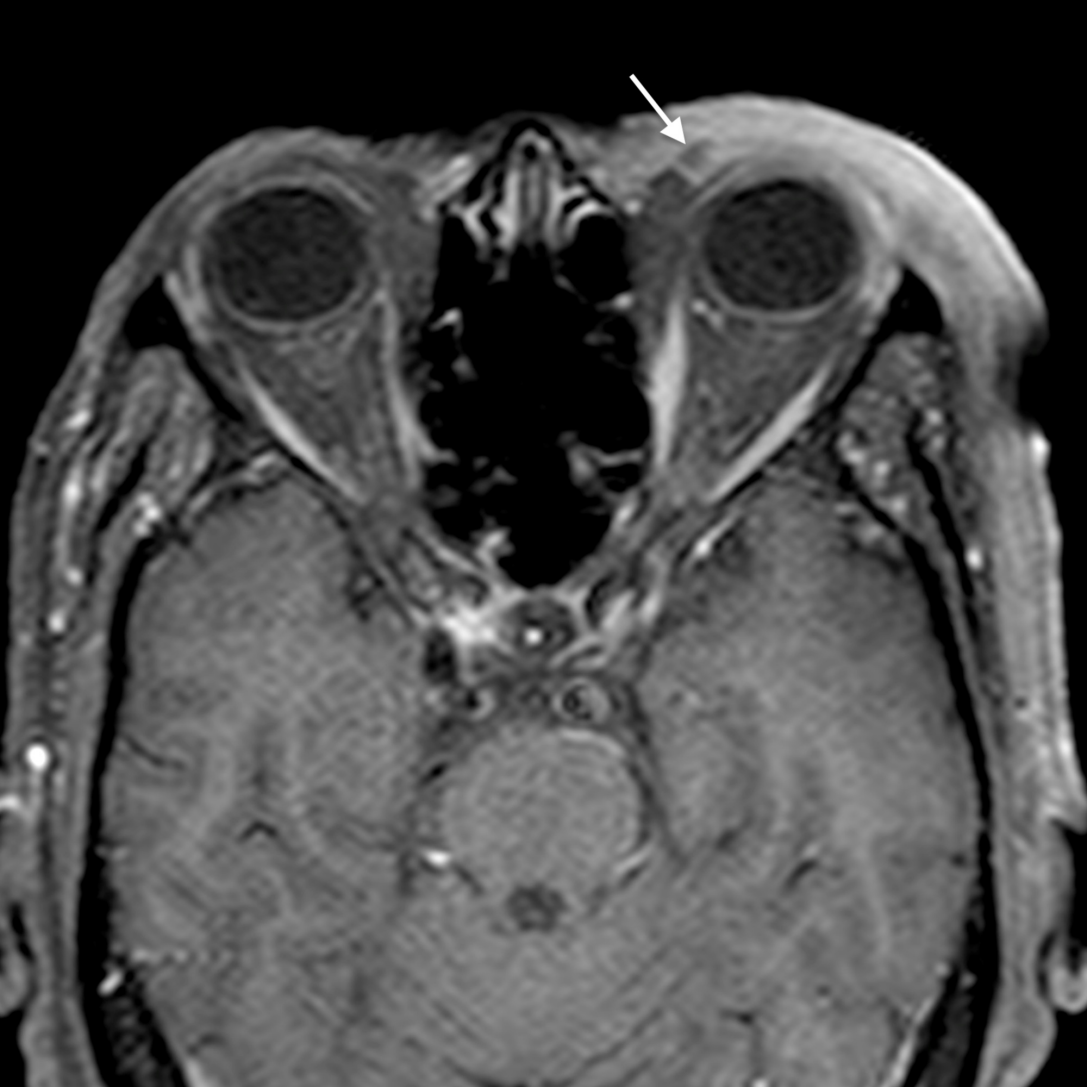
Preseptal cellulitis with abscess: axial post gadolinium T1WI shows diffuse enhancement in the preseptal space with a small peripherally enhancing area medially (arrow), indicative of preseptal abscess T1WI - T1-weighted image
